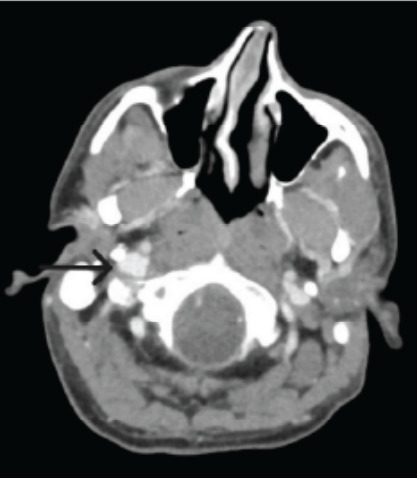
Axial computed tomography with a black arrow showing mild, asymmetric circumferential inflammation of the right carotid and extracranial internal carotid arteries.
